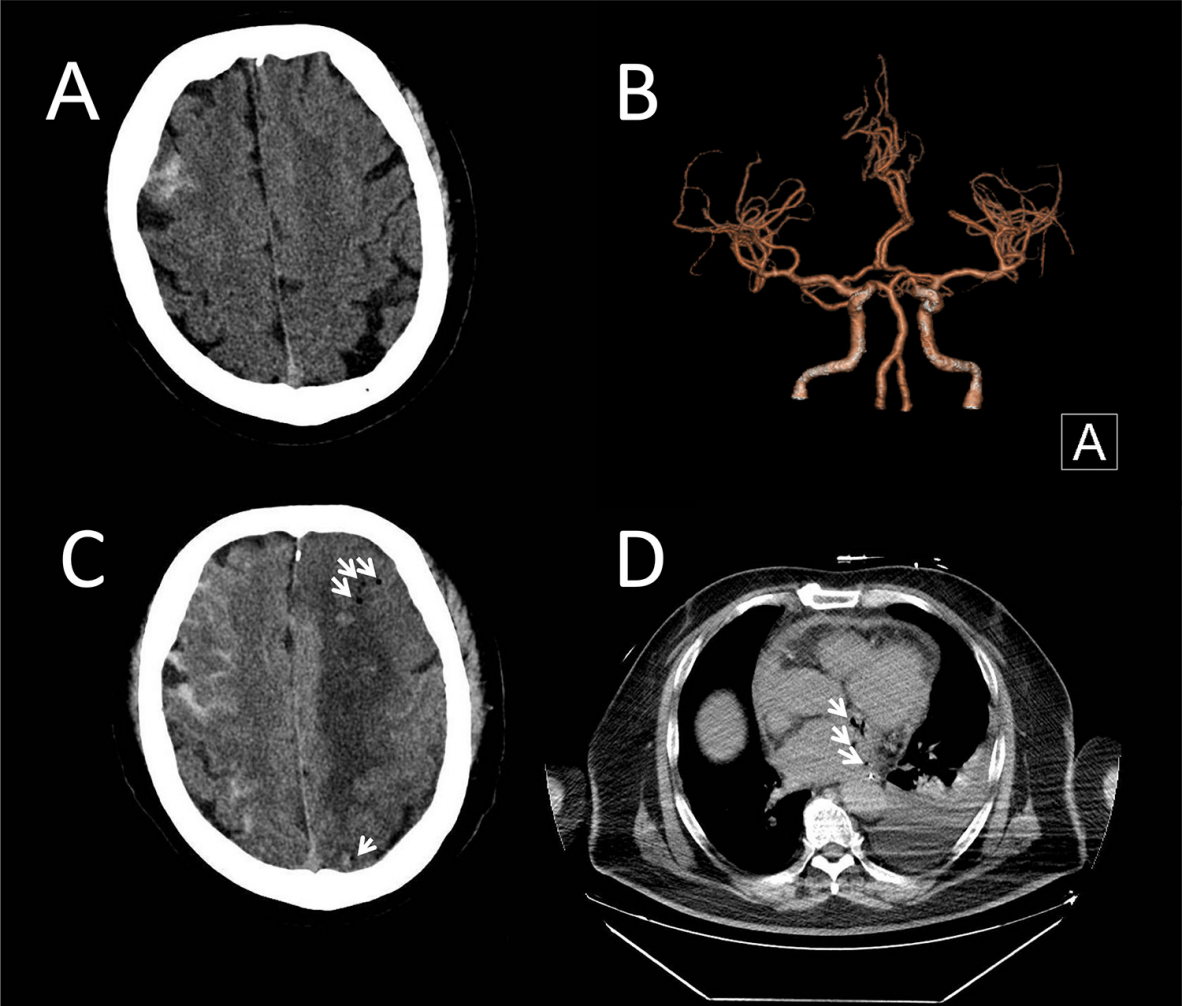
Brain CT and chest CT of the patient. (A) Initial cranial computed tomography (CT) revealed a right frontal subarachnoid hemorrhage. (B) CT angiography ruled out aneurysms. (C) Repeated cranial CT showed increased SAH, with air signals (arrows), infarcts and hemorrhagic densities in the left hemisphere. (D) Chest CT showed air signals (arrows) within and around the left atrium.
